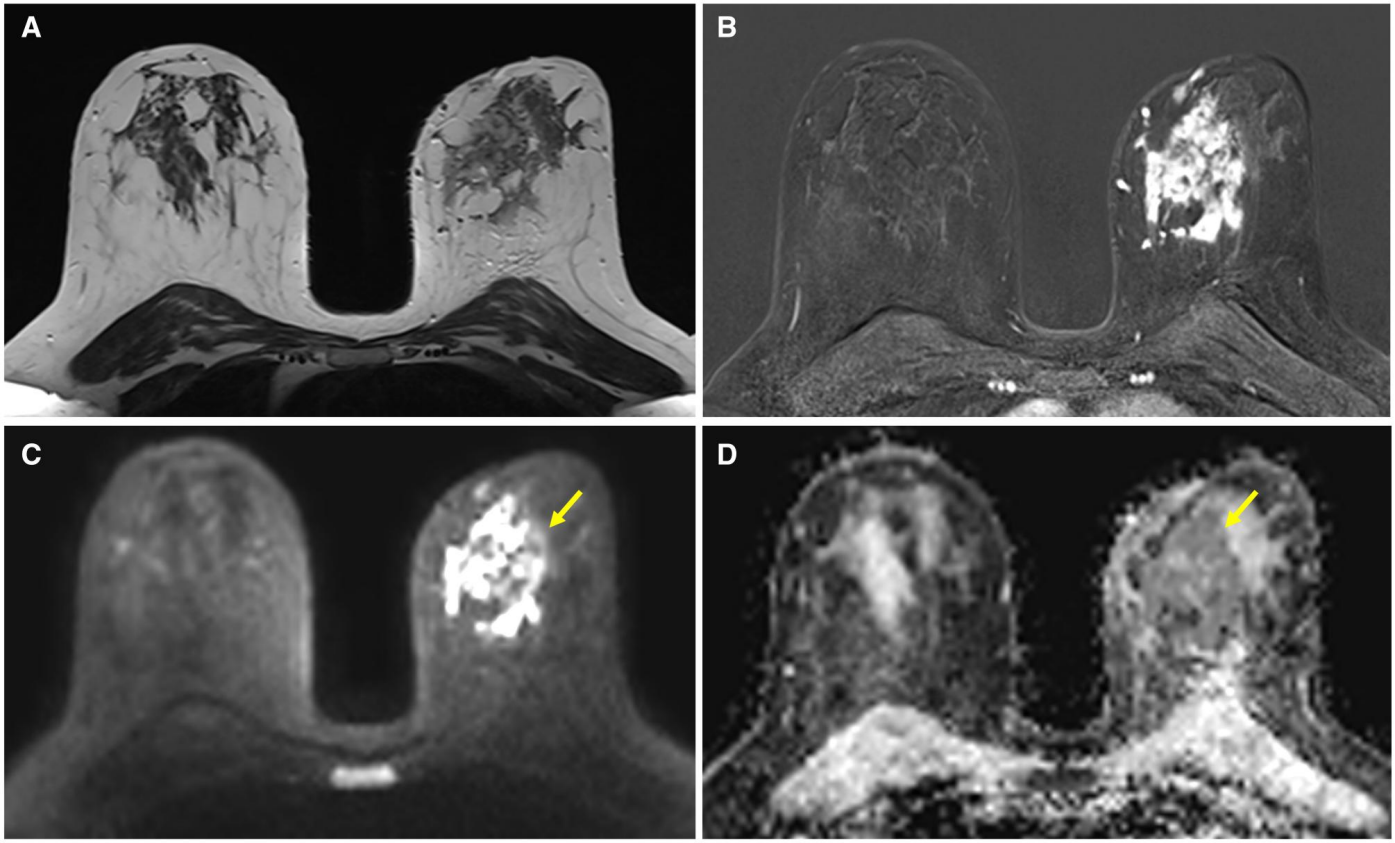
Breast MRI. (A) Axial T2-weighted, (B) axial contrast-enhanced T1-weighted spoiled gradient-echo (subtracted image obtained 120 s after contrast injection), (C) axial diffusion-weighted imaging (b-800 value), and (D) axial apparent diffusion coefficient (ADC) maps show an extensive area of heterogeneous non-mass-like enhancement in the internal quadrants of the left breast in multiple regions and restricted diffusion (arrows). ADC = 0.83 × 10^−3^ mm^2^/s. Note how MRI enables a better assessment of the overall extent of the lesion.
